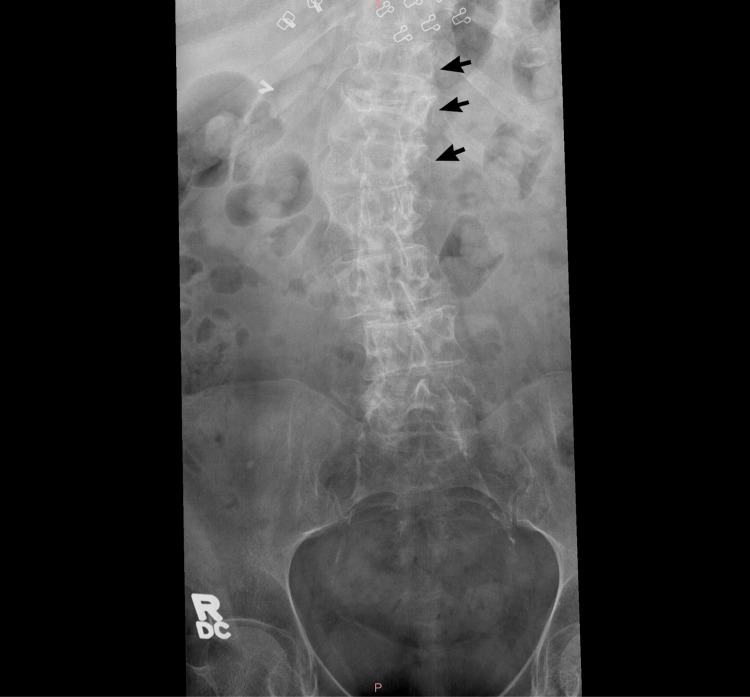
X-ray imaging showcasing compression fractures at T11, T12, and L1 levels, as well as degenerative scoliosis of the thoracolumbar spine
